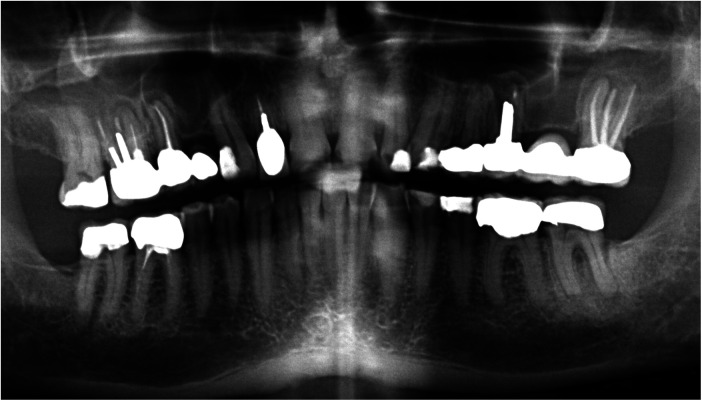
Orthopantomograph provided by the referring dentist at the time of patient referral to our clinic.
